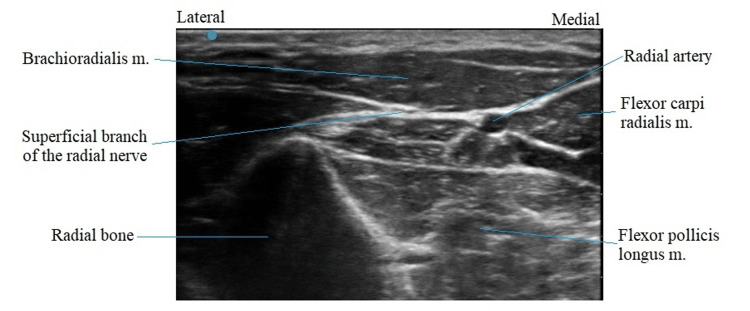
Ultrasonography of the superficial branch of the radial nerve m.: muscle
